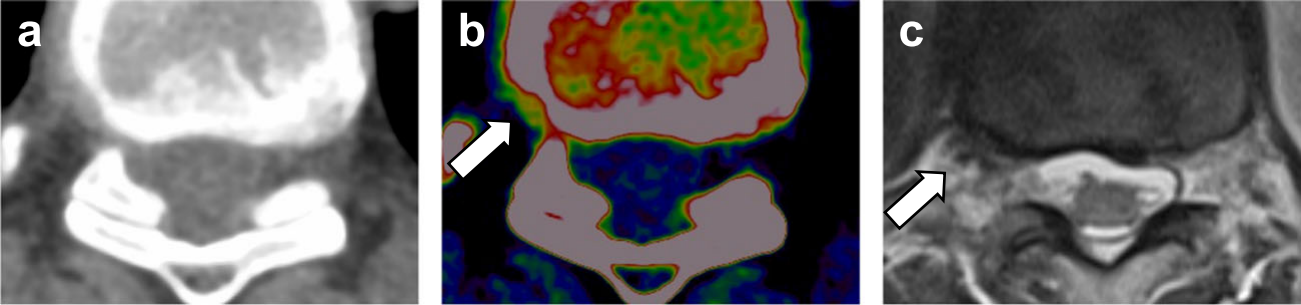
Case of a 67-year-old woman suffering from right-sided back pain who underwent spine dual-energy CT imaging. During analysis of transversal color-coded virtual noncalcium images (b), right-sided impingement of Th8 (contact, grade 1 according to the Pfirrmann nerve root compression grading system) due to disk bulging was detected by all readers in this study (*arrow*), which was initially missed on transversal grayscale CT series (a) by 3/5 readers. Additionally performed axial T2-weighted MRI series confirmed right-sided grade 1 spinal nerve root impingement (*arrow*) (c)
